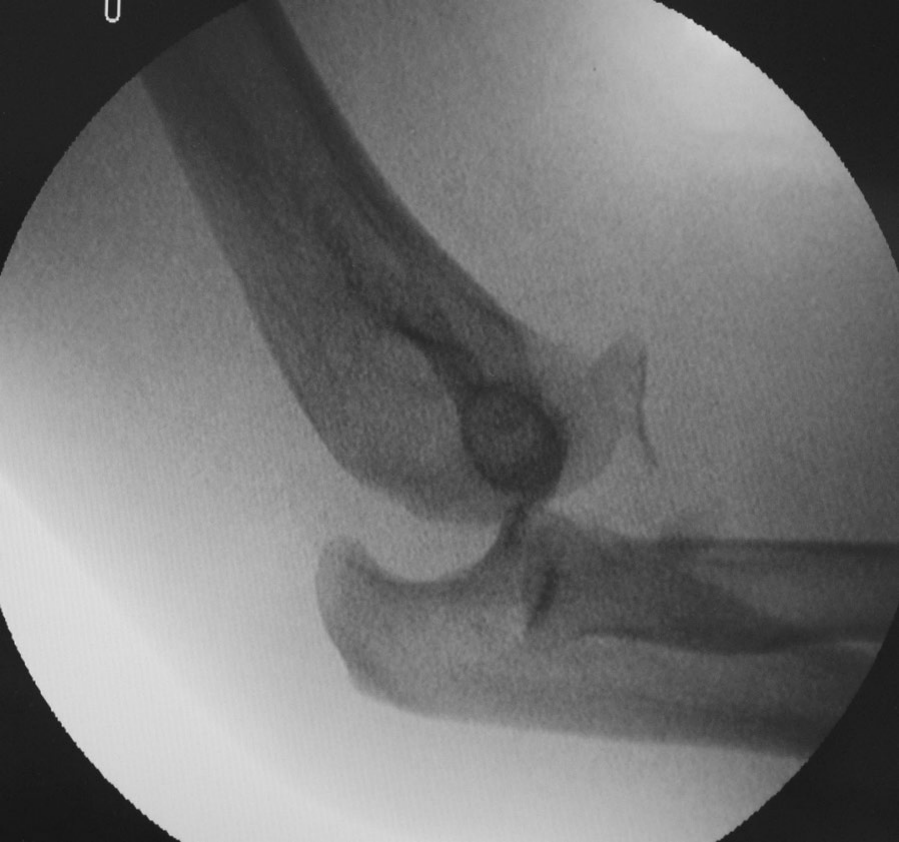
An example of instability defined by examination under anesthesia. A preoperative radiograph revealed posterior subluxation of the ulnohumeral joint during a test in a patient with terrible triad injury of the elbow
